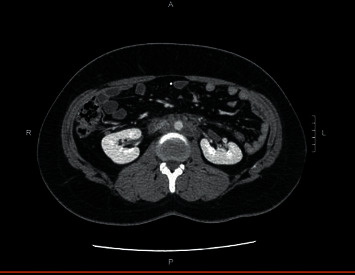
CT imaging of the abdomen and pelvis performed at four weeks shows improvement in retroperitoneal mass appearance.
